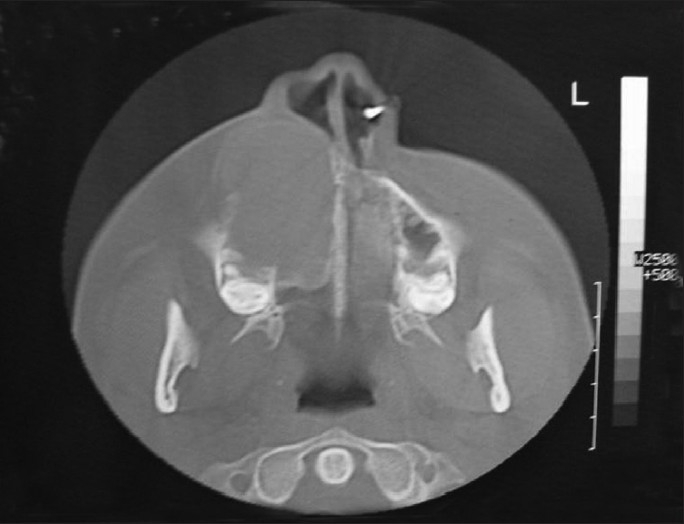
Axial computed tomography images showing expansion and thinning of the bony sinus wall that was absent at places
